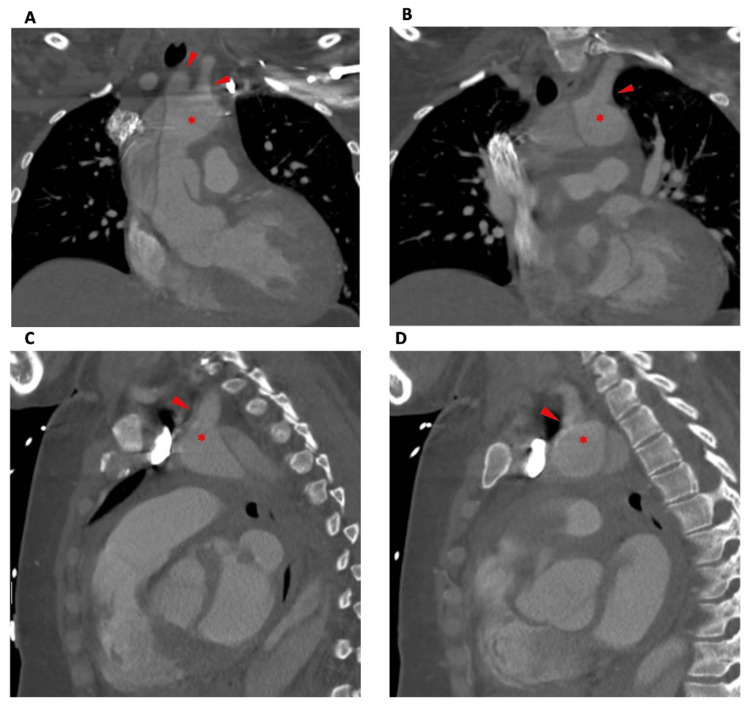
Type 1 aortic dissection with sparing of head vessels, seen on the CT scan on the day of admission Imaging shows the dissection beginning at the base of the ascending aorta and extending into the thoracic aortic arch, descending aorta, and the upper abdominal aorta. In the coronal series (A-B), the innominate and left common carotid arteries (A) and left subclavian (B) are shown. The dissection does not extend into these vessels. In the saggital series (C-D), the dissection can be clearly visualized distal to the left subclavian (C) and left common carotid artery (D); the dissection does not extend into these vessels (* indicates true lumen, red arrows indicate head vessels).
